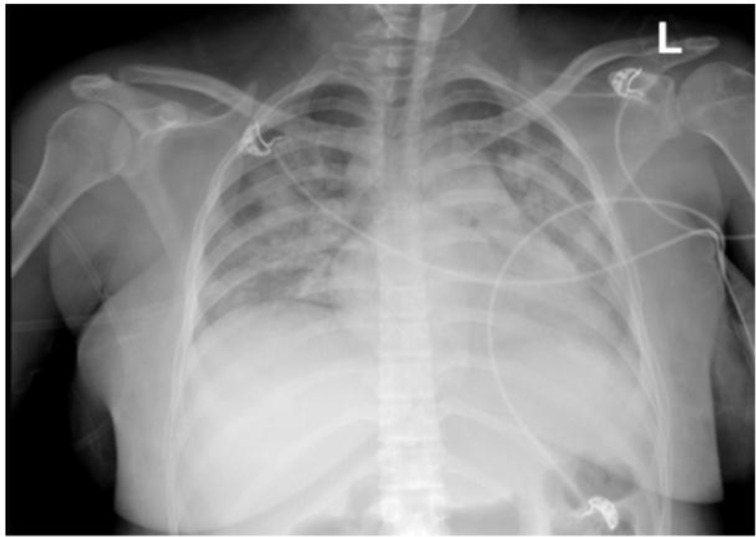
Chest X-ray revealing right lower lobe consolidation with extensive diffuse infiltrates.
